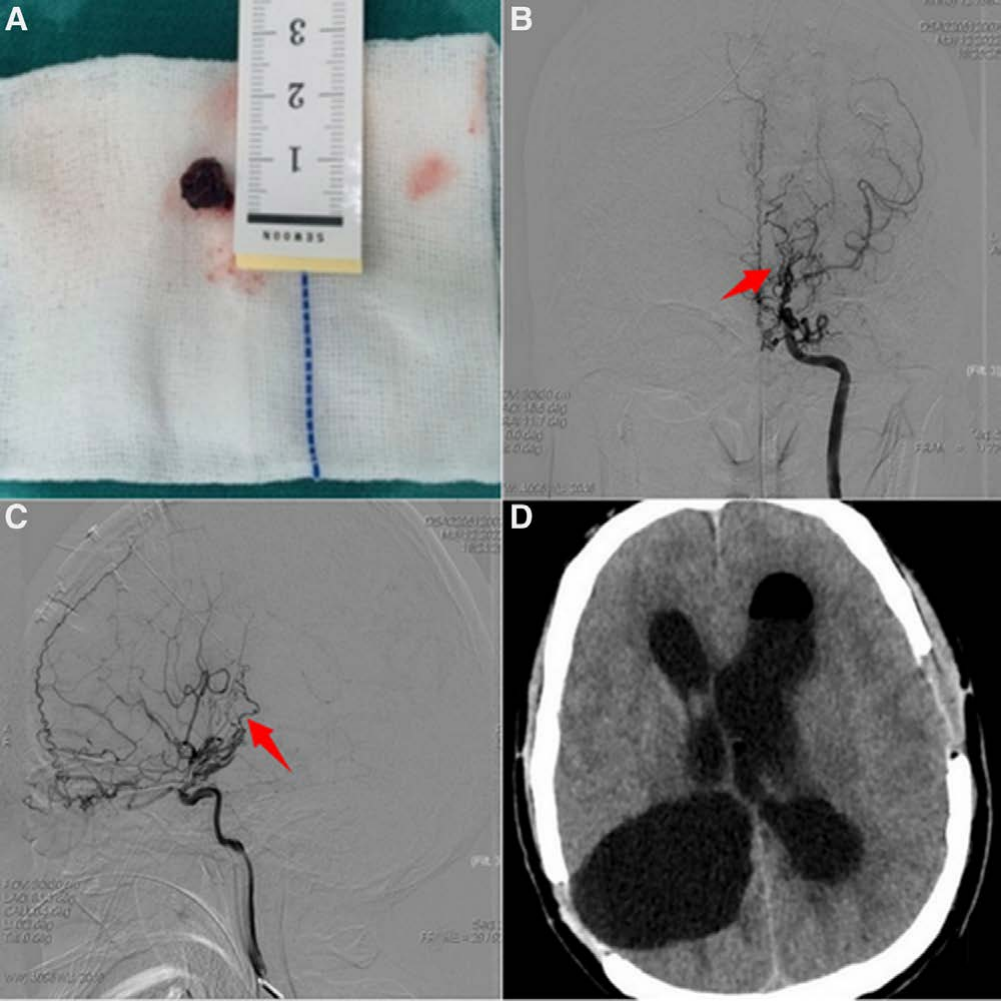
Postoperative imaging results of the patient. (A) is the aneurysm removed by craniotomy. (B,C) are cerebral vascular images taken during DSA of the patient’s brain after craniotomy, showing that the aneurysm has dissipated (red arrows mark the area of prior aneurysm), the anterior choroidal artery opacifies normally. (D) is a CT of the head taken before the patient was discharged, demonstrating the complete disappearance of the intracerebroventricular hematoma. CT = computerized tomography, DSA = digital subtraction angiography.
